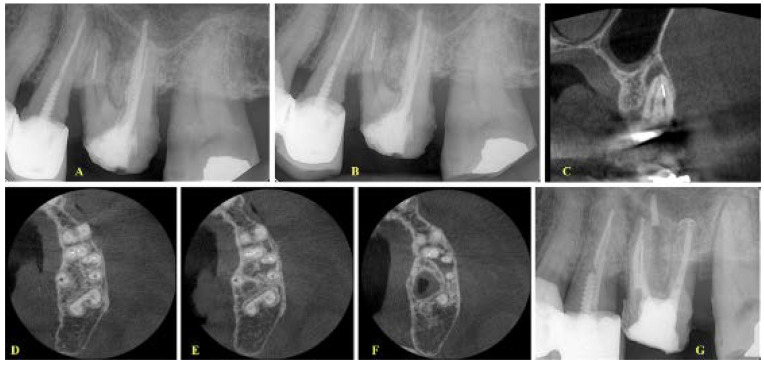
(A) Initial radiograph of a maxillary first molar with periapical lesions and an instrument fracture at the middle-apical third of the mesial root, (B) A second initial radiograph (mesial view) of the case, (C) Coronal view demonstrating that the fragment is located at the mesiobuccal 1 canal (MB1) and the two canals are merging at the apical third, (D–F) Consecutive axial views demonstrating that the two canals have a common apical foramen, (G) Final radiograph with the fragment in situ. No effort was made for the removal of the fragment. Apical patency was achieved through the mesiobuccal 2 canal (MB2).
